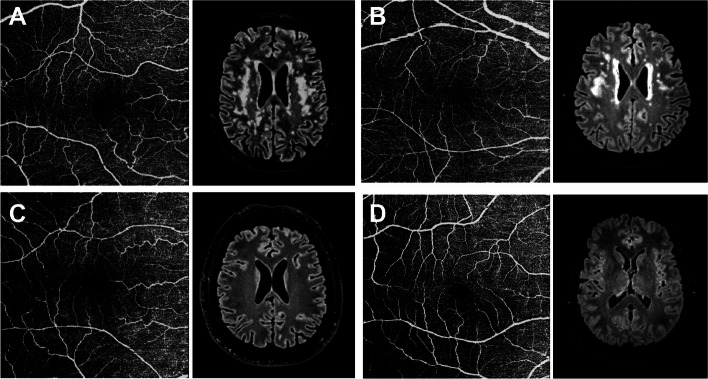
Representative OCT-A and brain MRI images from the study participants. A 68-year-old female with SCD and high CV damage (Fazekas scale score = 2, WMH volume = 30,943 mm^3^). B 61-year-old female with MCI and high CV damage (Fazekas scale score = 3, WMH volume = 27,000 mm^3^). C 61-year-old female with SCD and low CV damage (Fazekas scale score = 0, WMH volume = 290 mm^*3*^). D 60-year-old female with MCI and low CV damage (Fazekas scale score = 0, WMH volume = 710 mm^3^). Abbreviations: CV: cerebrovascular; MCI: mild cognitive impairment; MRI: magnetic ressonance imaging; OCT-A: Optical Coherence Tomography – angiography; SCD: subjective cognitive decline; WMH: white matter hyperintensity
